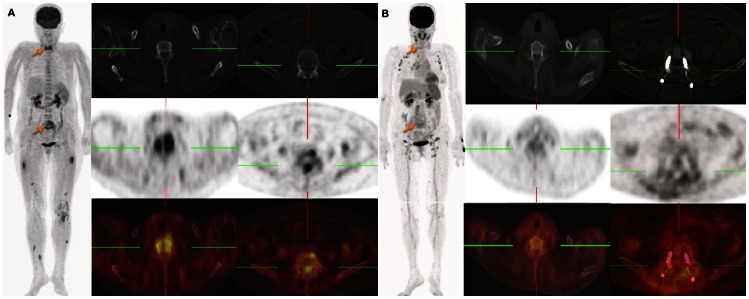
A 60 year-old male admitted with a diagnosis of *Staphylococcus aureus* sepsis of unknown origin. (A) The maximum intensity projection of PET and the transaxial views of CT, PET, and fused PET/CT images revealed an increased FDG uptake at C6 and L5-S1 in the spine (arrow). Subsequent MRI imaging confirmed the diagnosis of infectious spondylitis. Several metastatic foci were also bilaterally evident in the thighs and calves. (B) The patient underwent laminectomy at L4-S1 and interbody fusion. The pathological results confirmed the presence of necrotic bone and fibrous tissue to form an abscess. The results of follow-up imaging at 6 weeks showed the resolution of spine osteomyelitis (arrow). FDG PET/CT imaging demonstrated soft tissue inflammation in the postsacral region, as well as the presence of axillary and inguinal lymphadenopathy due to reactive hyperplasia.
